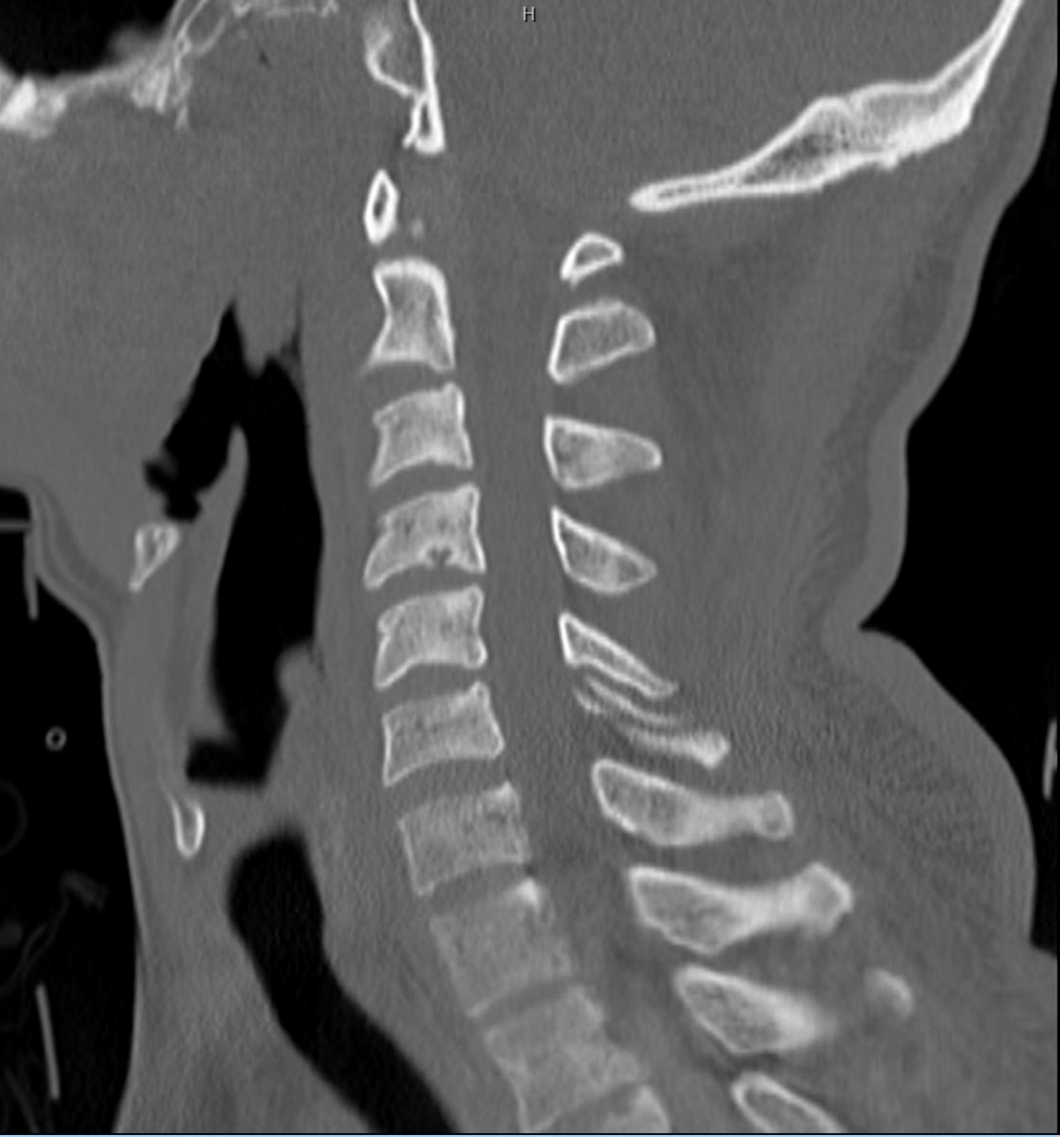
Computed Tomography Scan of the Cervical Spine Midline sagittal imaging that shows a lack of anterolisthesis
